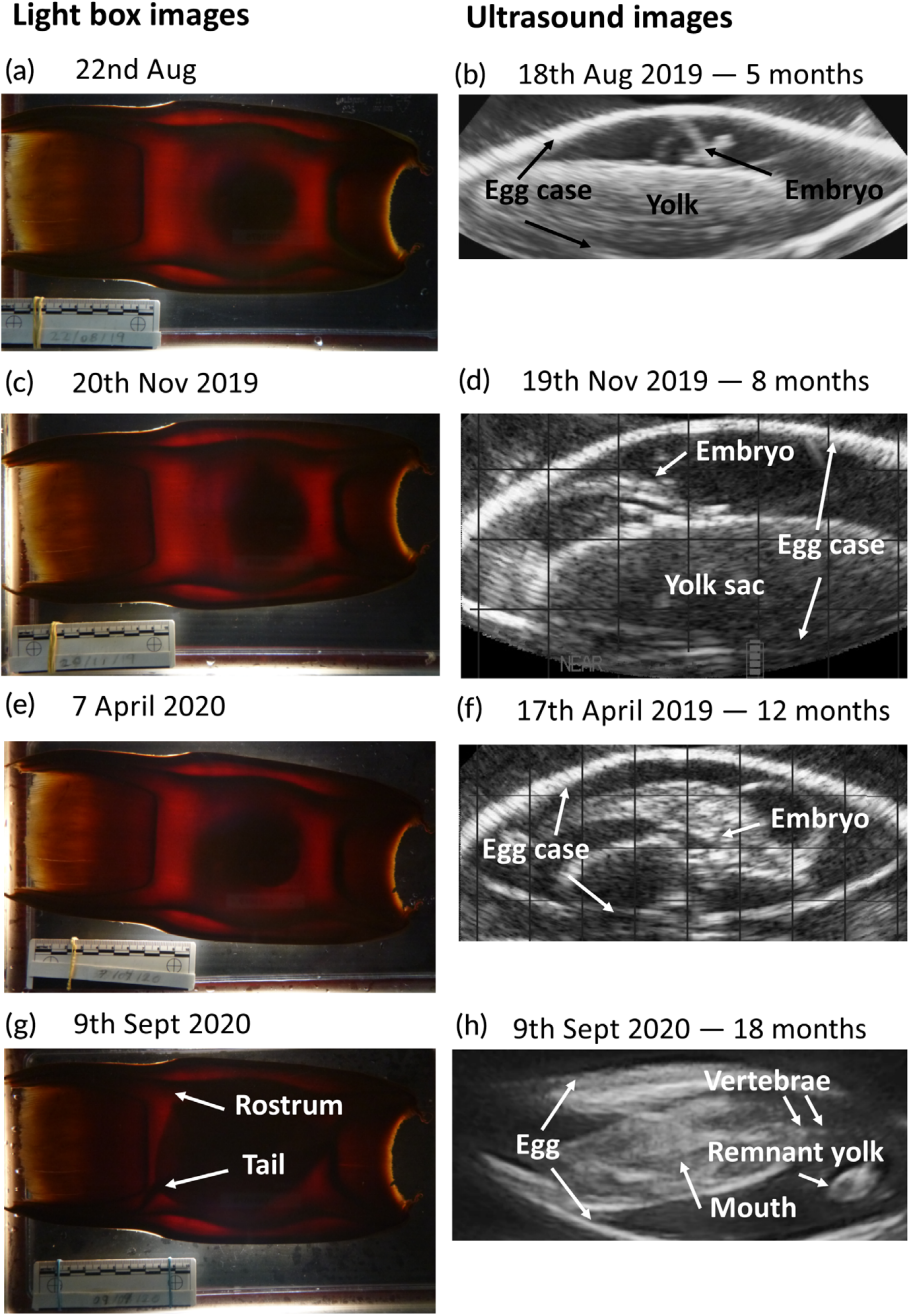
Light box and ultrasound images at 5, 8, 12 and 18 month development. Gridlines (where included) represent 10 mm × 10 mm. (a) Image recorded when egg was photographed on 22nd Aug 2019. (b) Ultrasound image of egg recorded on 18th Aug 2019, 5 months after laying. (c) Image recorded when egg was photographed on 20th Nov 2019. (d) Ultrasound image of egg recorded on 19th Nov 2019, 8 months after laying. (e) Image recorded when egg was photographed on 7th April 2020. (f) Ultrasound image of egg recorded on 17th April 2020, 12 months after laying. (g) Image recorded when egg was photographed on 9th Sept 2020. (h) Ultrasound image of egg recorded on 9th Sept 2020, 18 months after laying
